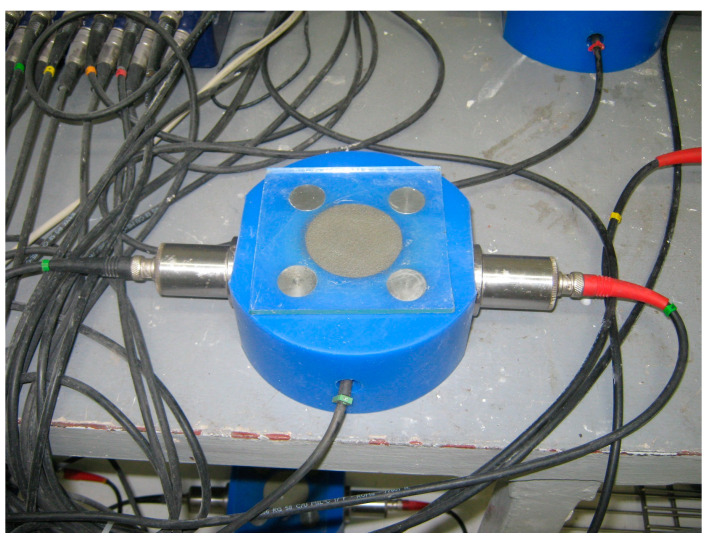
Mortar sample during ultrasonic testing.
